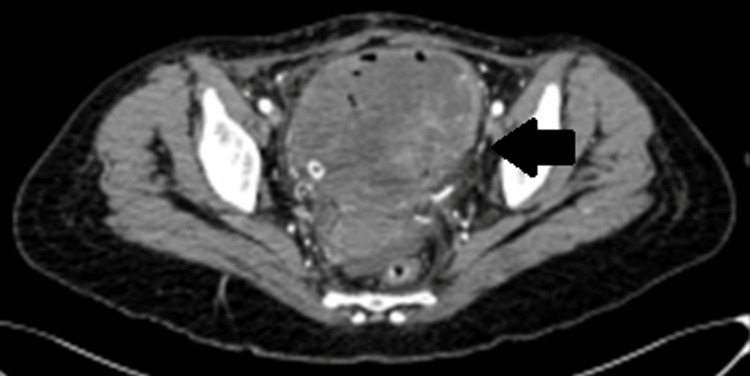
CT angiogram showing increase in the large irregular soft tissue density mass seen rising from the left lateral wall and base of the bladder, currently measuring 10 x 9.0 x 9.0 cm, while previously measuring 6.1 x 6.0 x 6.0 cm. CT, computed tomography
